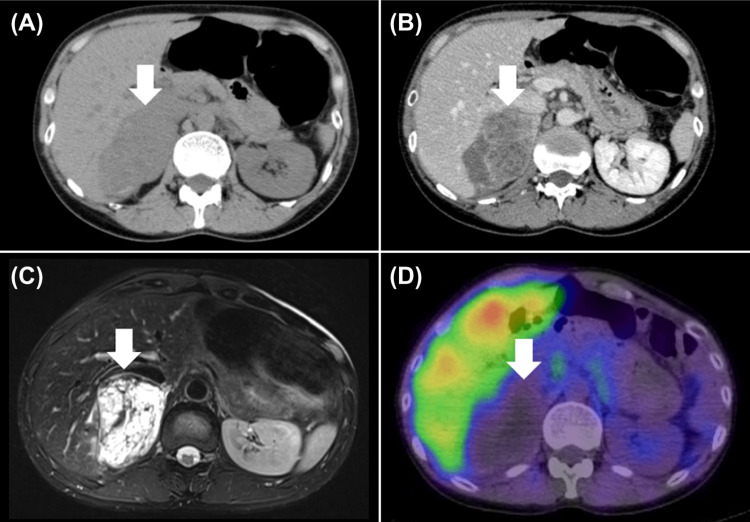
Imaging examination of the right adrenal tumor in case 38. (A) Plain CT. (B) Contrast-enhanced CT. (C) T2-weighted MRI. (D) 123I-MIBG scintigraphy. (A) Unenhanced CT image showing a right adrenal mass. (B) Contrast-enhanced CT with moderate enhancement of the tumor. (C) T2-weighted MRI showing marked hyperintensity. (D) ¹²³I-MIBG scintigraphy showing negative uptake in the right adrenal gland. ^123^I-MIBG: ^123^I-metaiodobenzylguanidine.
